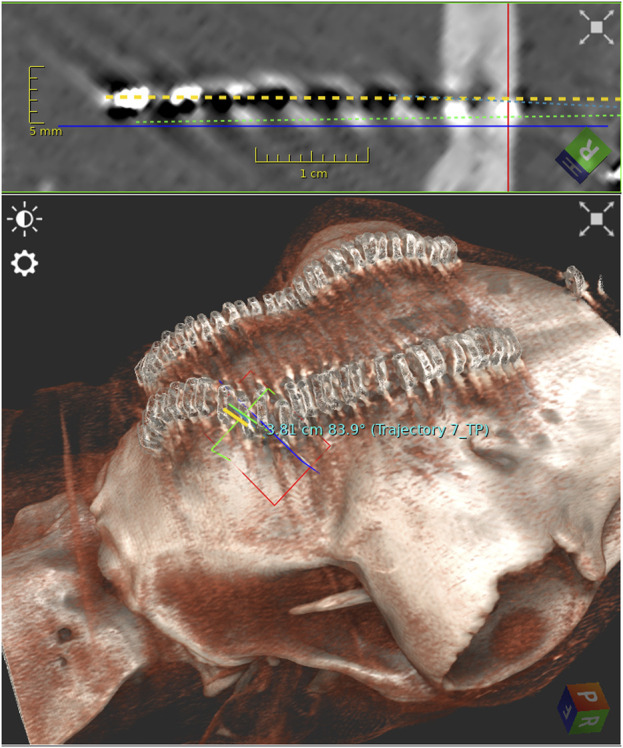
Postoperative computed tomography imaging with 3-dimensional reconstruction showing the planned trajectory and depth electrode alignment.
